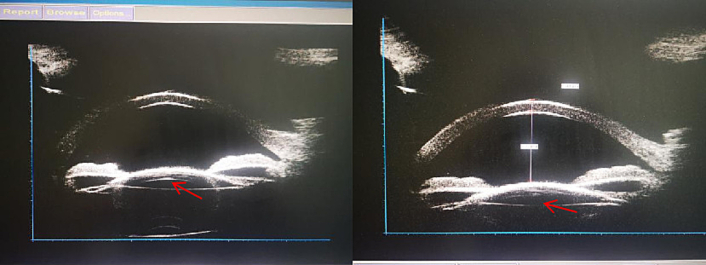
Preoperative ultrasonography of the UBM examination. Photo of the intraocular slit lamp of the invisible intraocular lens.
